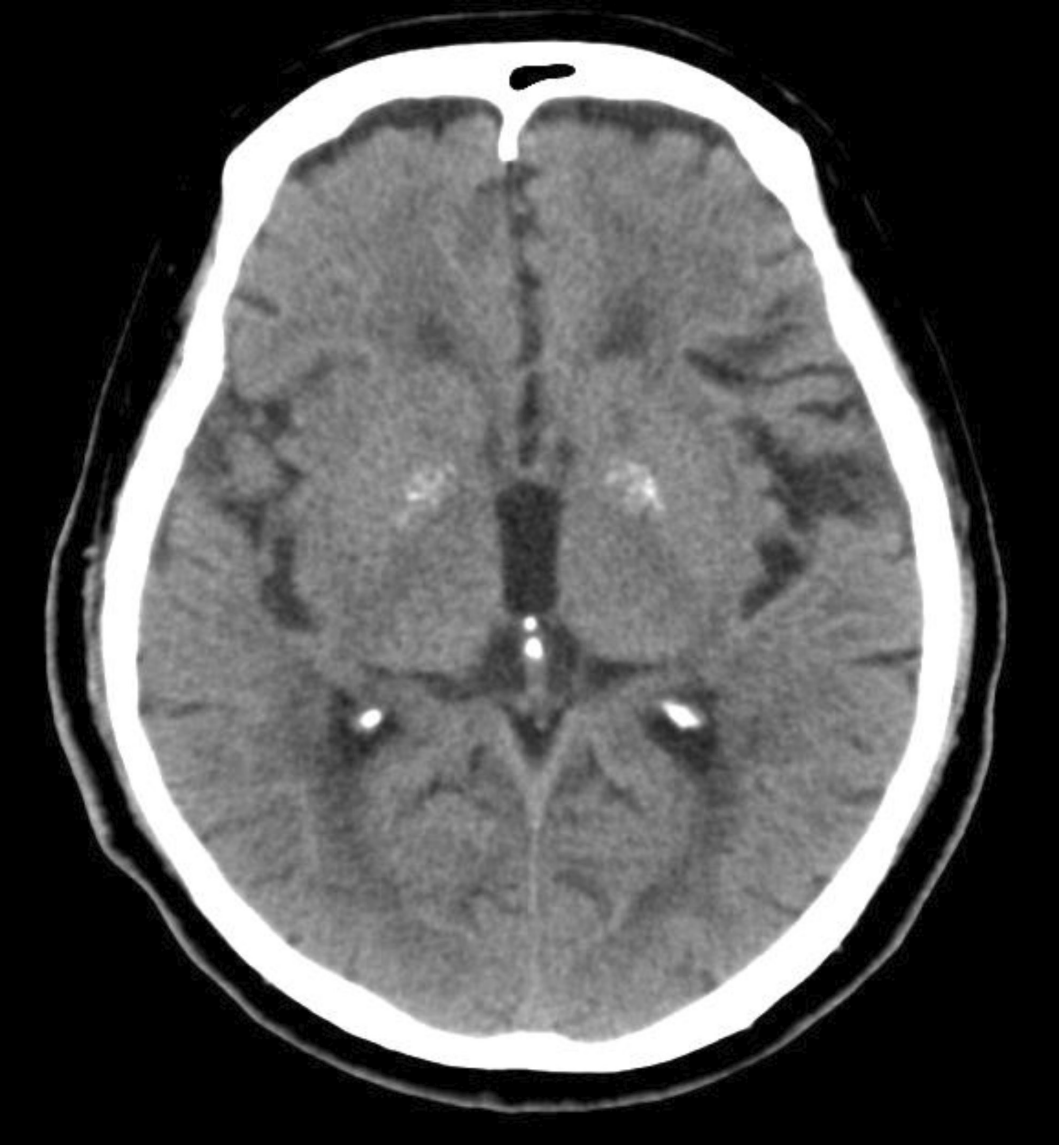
Head computed tomography: There was no evidence of intracranial hemorrhage.
